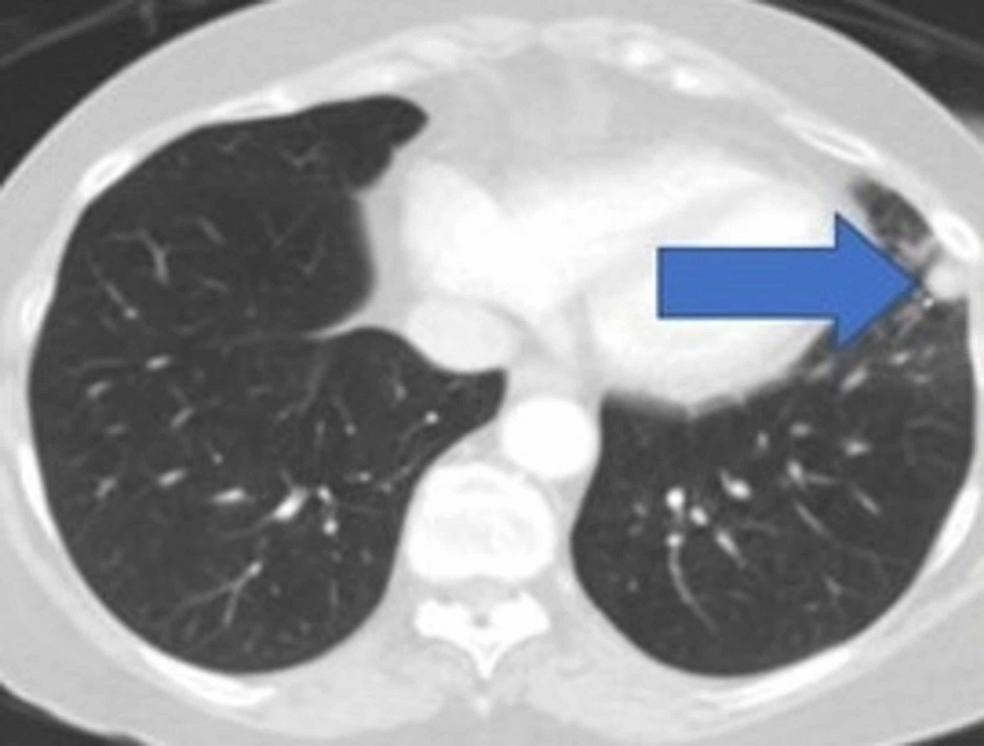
Chest CT. Arrow identifying left lower lobe nodule CT: computed tomography
